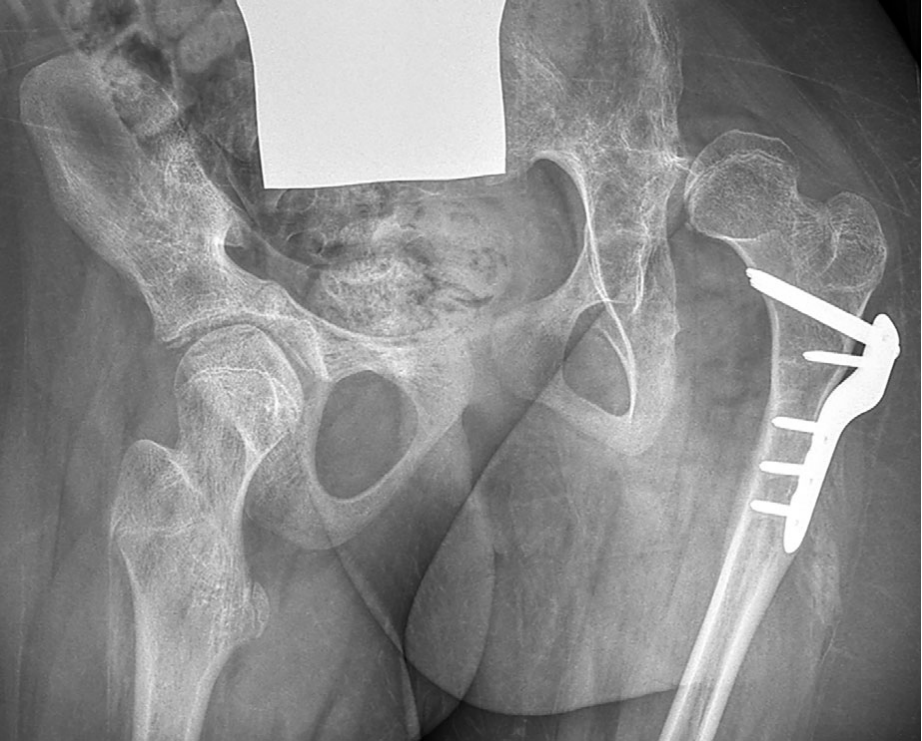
Radiograph of a 14‐year‐old female with bilateral spastic cerebral palsy in Gross Motor Function Classification System level IV, showing severe subluxation and femoral head deformity of the left hip and marked pelvic obliquity. She had unilateral severe pain in her left hip (Child Health Questionnaire hip pain score 20).
